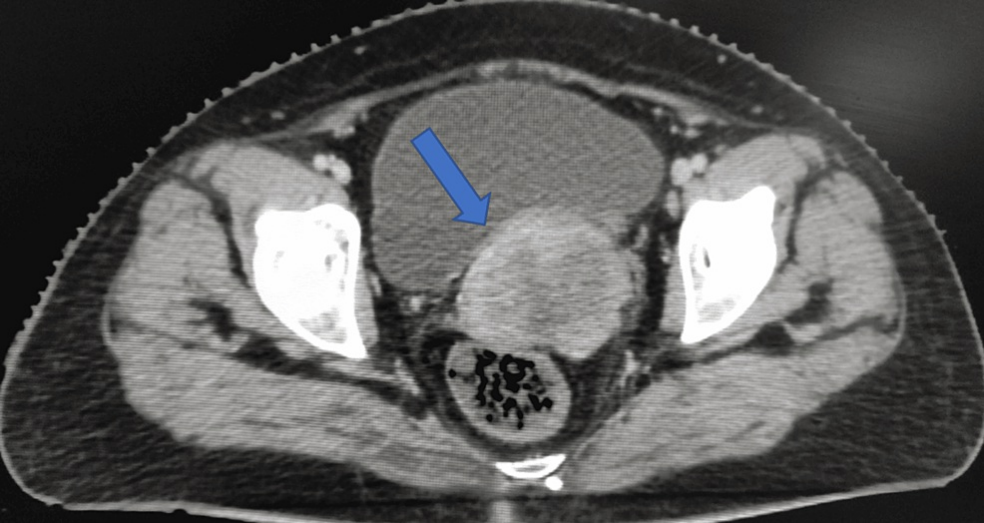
Axial section of the CECT of the pelvis showing the 6 cm × 7.5 cm × 9 cm lesion involving the cervix. CECT: contrast-enhanced computed tomography
